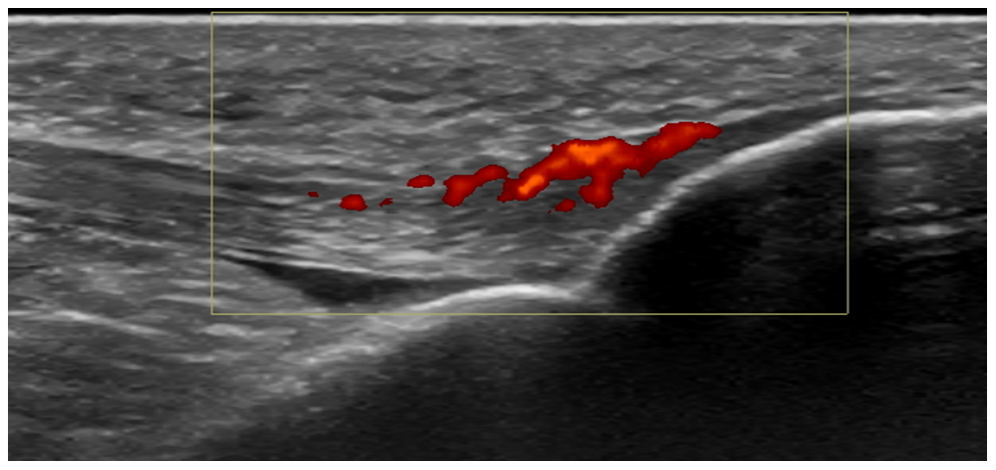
Positive power Doppler signal identifying tibial tuberosity enthesitis. The signal (in red) is detected in the tibial insertion of the patellar ligament in one of the studied patients.
